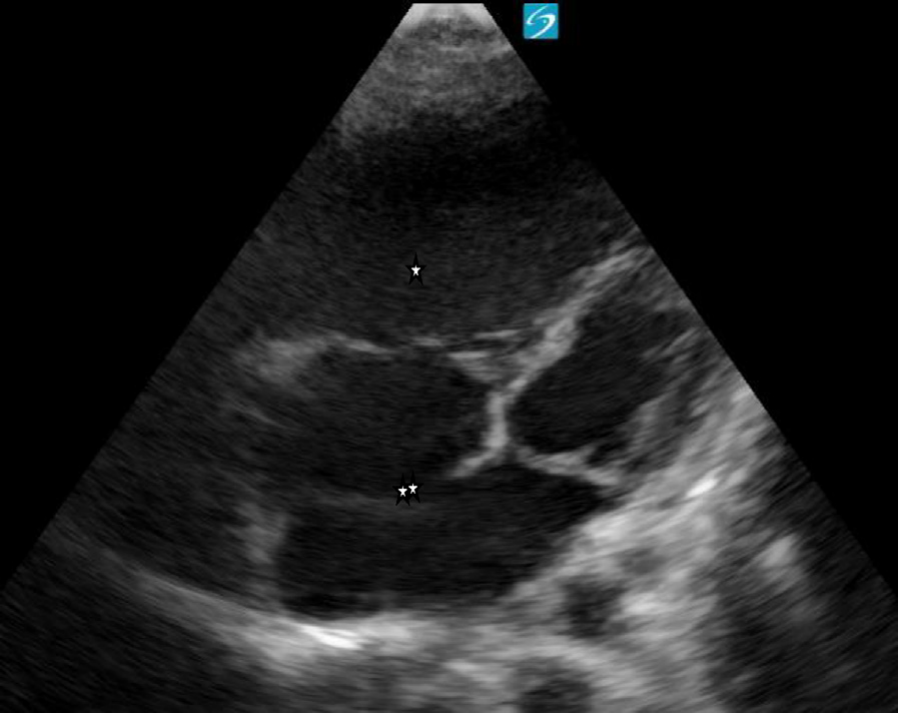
Apical four-chamber view on point-of-care ultrasound revealing dilation of the right atrium and right ventricle (∗), as well as an atrial septal defect (∗∗). Image taken by the author.
